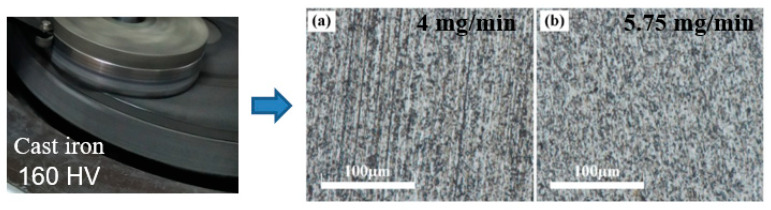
Optical images after lapping using cast iron pad: (a) common lapping; (b) ultrasonic-assisted lapping.
